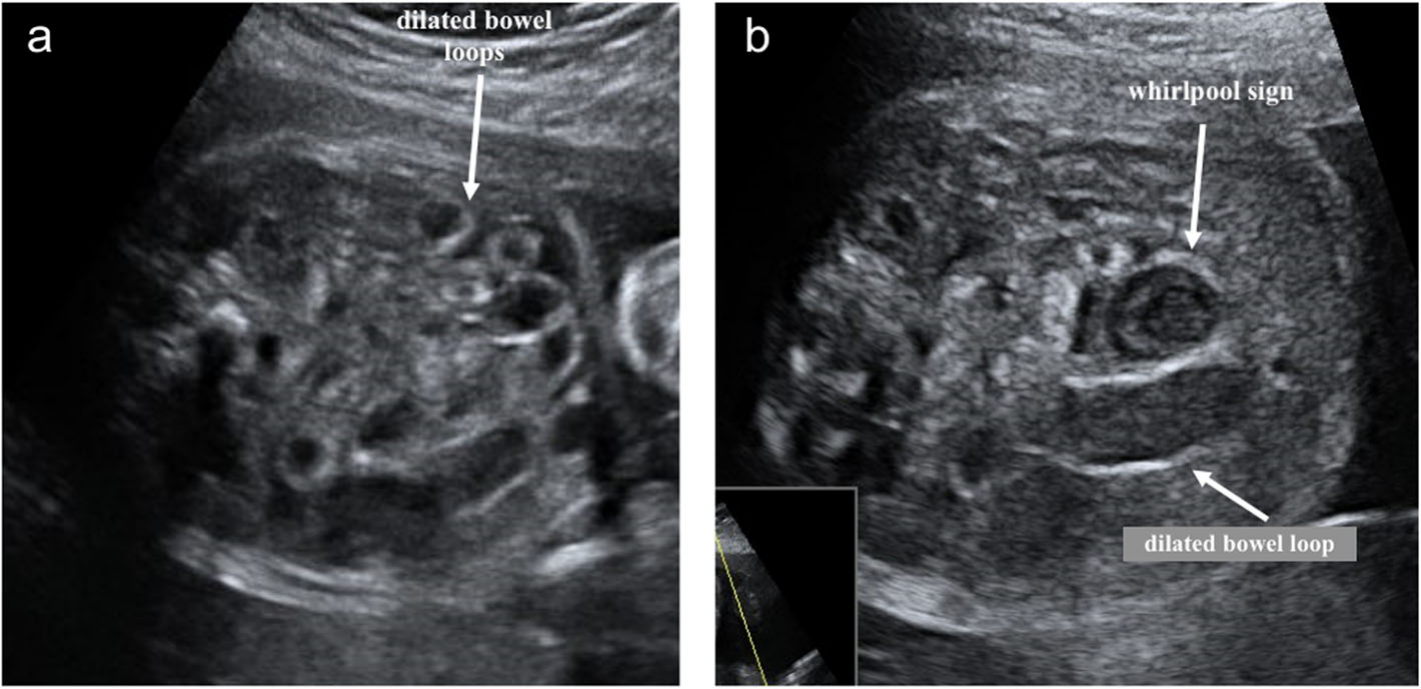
Ultrasound image of fetal volvulus in pump twin. Transverse view of the fetal abdomen with *white arrows* pointing to the (a) dilated bowel loop segments and (b) dilated bowel loop in a whirlpool configuration
